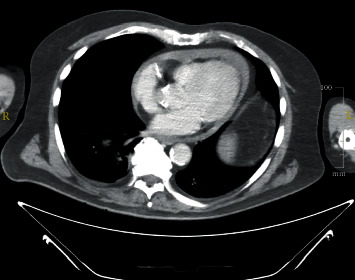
CT scan showed the presence of global pericardial effusion.
